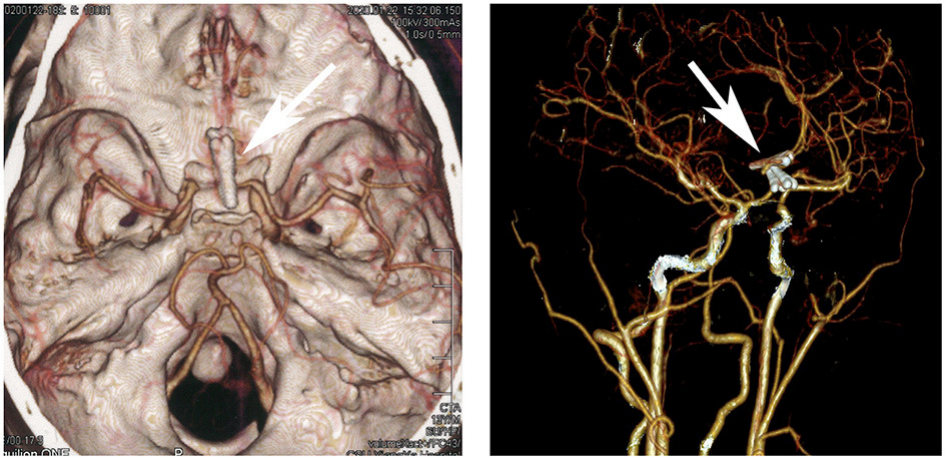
Cerebral computed tomography angiography showing the ruptured anterior cerebral aneurysm (arrow).
